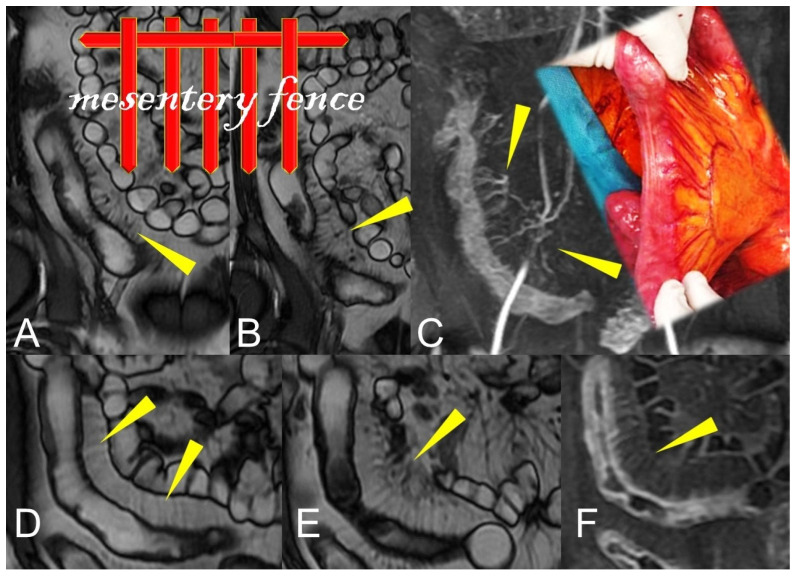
During the active phase, the vascularization of the mesenteric fan increases with an evident engorgement of the vasa recta that takes on the appearance of a clear palisade that stands out against the background of the hypertrophic mesentery. According to some, this aspect commonly resembles the teeth of a hair comb (comb sign) but which we like to imagine instead, due to the thickness of the hypertrophic vasa recta, as a palisade of a fence (mesentery fence). (A,B,D,E): Coronal fast imaging employing steady-state acquisition (FIESTA) image, (C): coronal contrast-enhanced fat-suppressed T1-weighted maximum intensity projection image, and (F): coronal contrast-enhanced fat-suppressed T1-weighted image show engorgement of the vasa recta (yellow arrowheads in (A–F) images.
